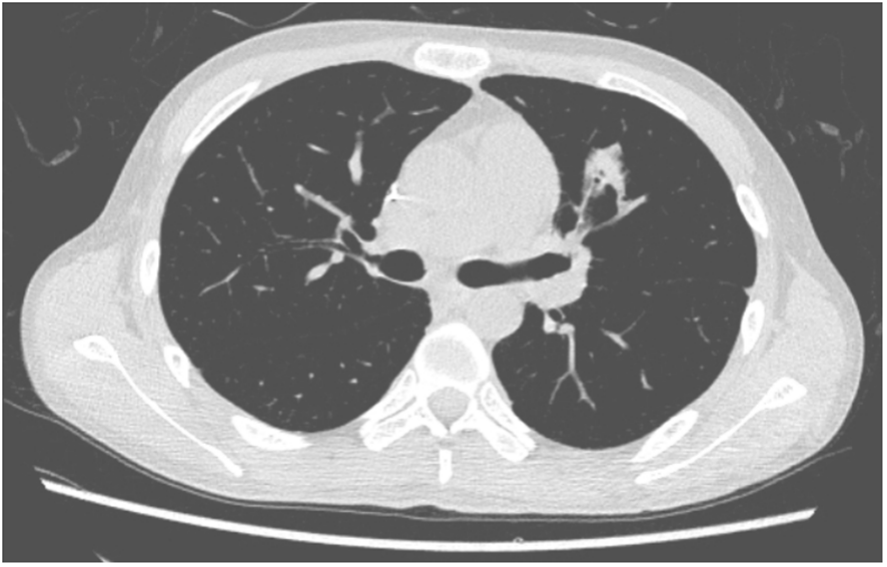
Case 2 lung CT on day +31.
